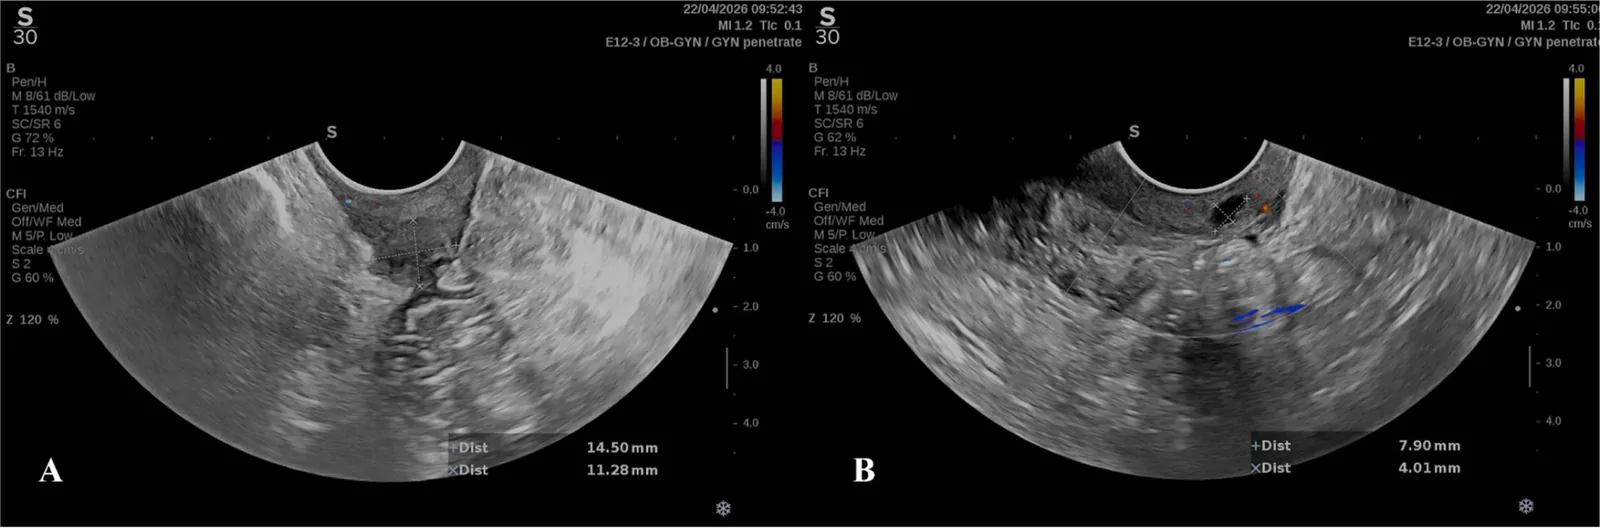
Transvaginal ultrasound images of a 46-year-old woman 12 months after total laparoscopic hysterectomy with ovarian preservation. (A) Hypoechoic nodule at the vaginal cuff measuring 14.5 × 11.3 mm (calipers), suggestive of post-surgical changes or small vaginal myoma. (B) Thin-walled cyst in the anterior upper vaginal wall measuring 7.9 × 4.0 mm (calipers). Both ovaries are normal in size and echogenicity with preserved mobility. Mild free fluid is present in the pelvic cavity.
